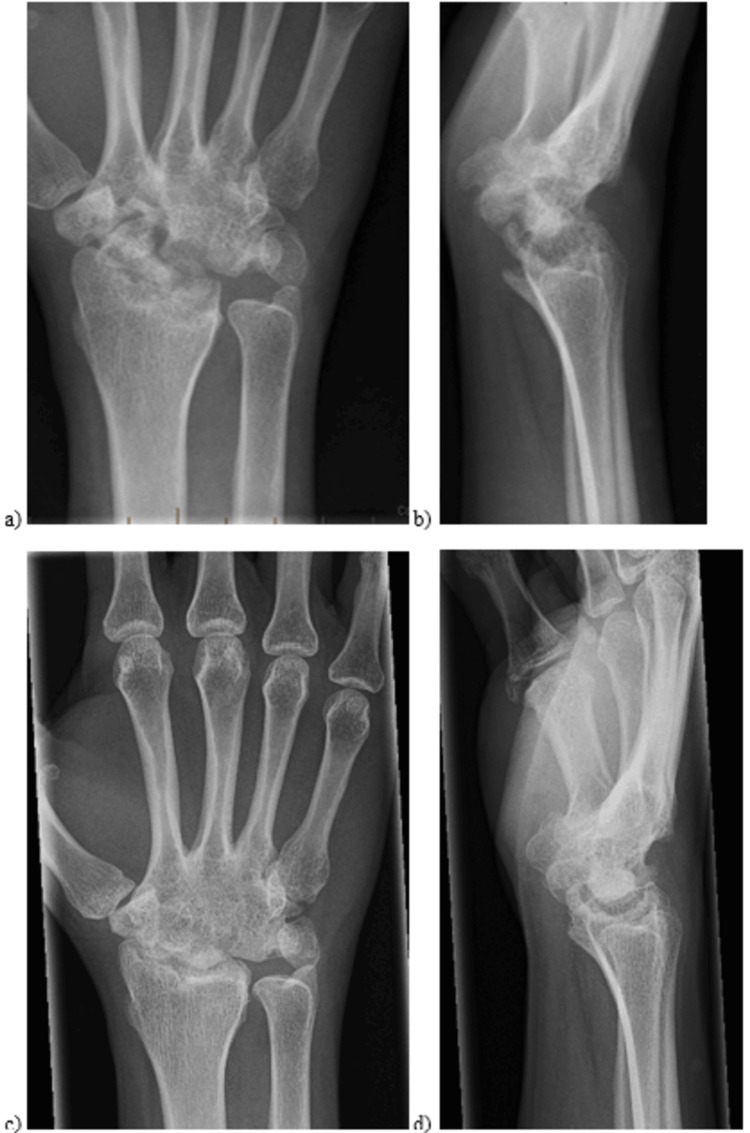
Radiographic follow-up at one year and eight years showing osseous remodeling with osseous fusion at the intercarpal bones and carpometacarpal joint with degenerative changes of the radiocarpal joint. Radiograph at the one-year follow-up: a) anteroposterior view and b) lateral view. Radiograph at the eight-year follow-up: c) anteroposterior view and d) lateral view.
